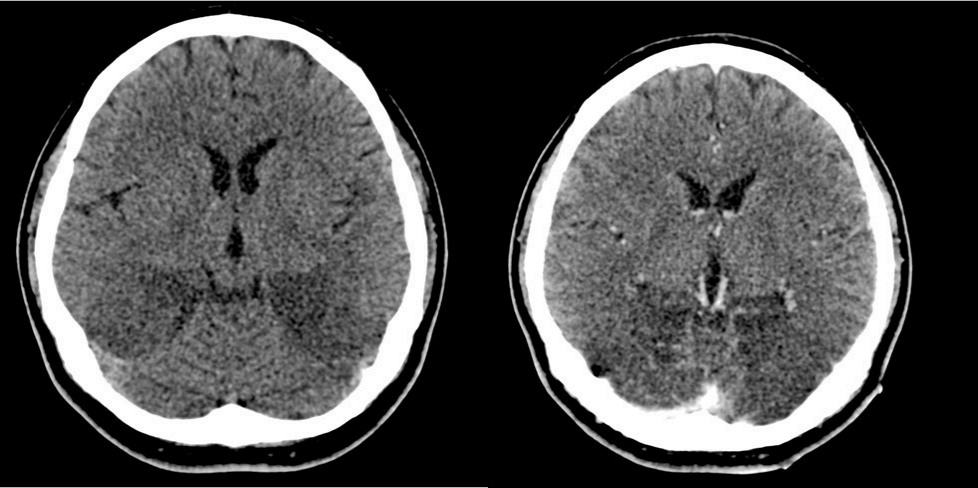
Plain (top left) and contrasted (top right) CT scans show progressive/enlarging hypodense encephalitic changes in both occipital lobes.
